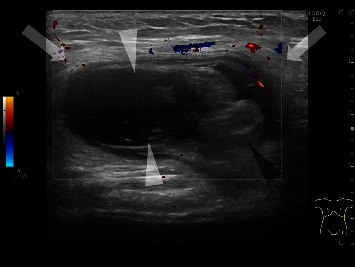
Case 3: a 7-year-old boy with undescended testicular torsion. Sonogram of the left inguinal bulge shows a low-echoic testis (white arrowheads) accompanied by swelling of the epididymis (black arrowhead). This low-echoic structure was not connected to the abdomen and was not detected to be a layer of the intestinal wall. Vascular perfusion within the testis and epididymis is diminished. The surrounding subcutaneous tissue exhibits edematous changes (arrows).
